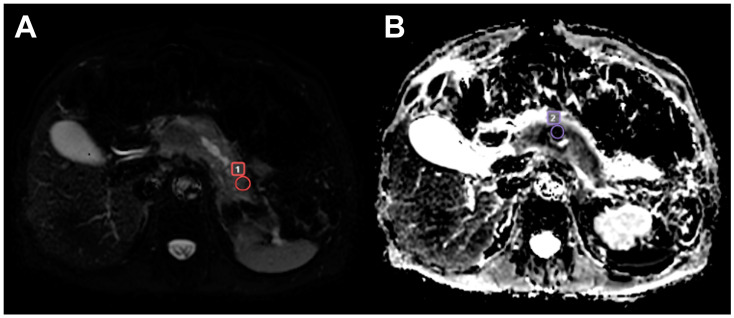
ROI placement in a bifocal ductal adenocarcinoma of the pancreas: (A) a DWI image at b0 with an ROI in the tail tumor (1); and (B) an ADC image with an ROI in the trunk tumor (2).
